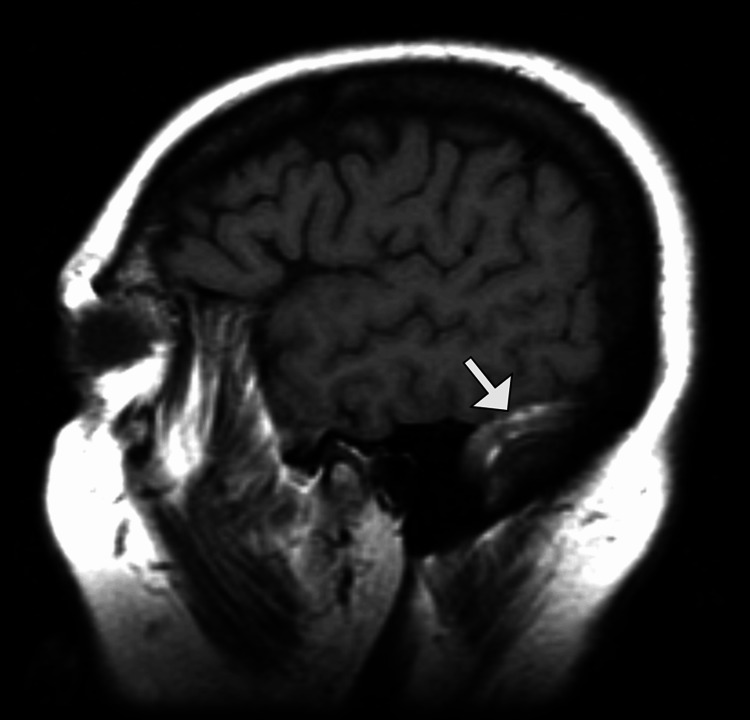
T1-weighted MRI showing thrombus within the transverse sinus Sagittal T1-weighted magnetic resonance imaging (MRI) demonstrates abnormally high signal intensity within the right transverse sinus (arrow), compatible with a subacute thrombus.
